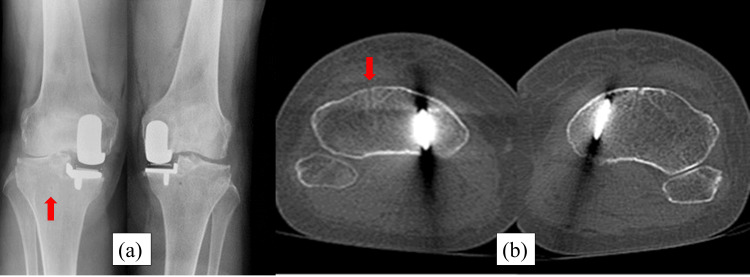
Immediately postoperative radiography and computed tomography (CT). (a) Postoperative radiography shows no fracture line. (b) Postoperative CT shows the level of a pinhole. The red arrows show the place of the pin. The pin was placed outside the tibial mechanical axis.
